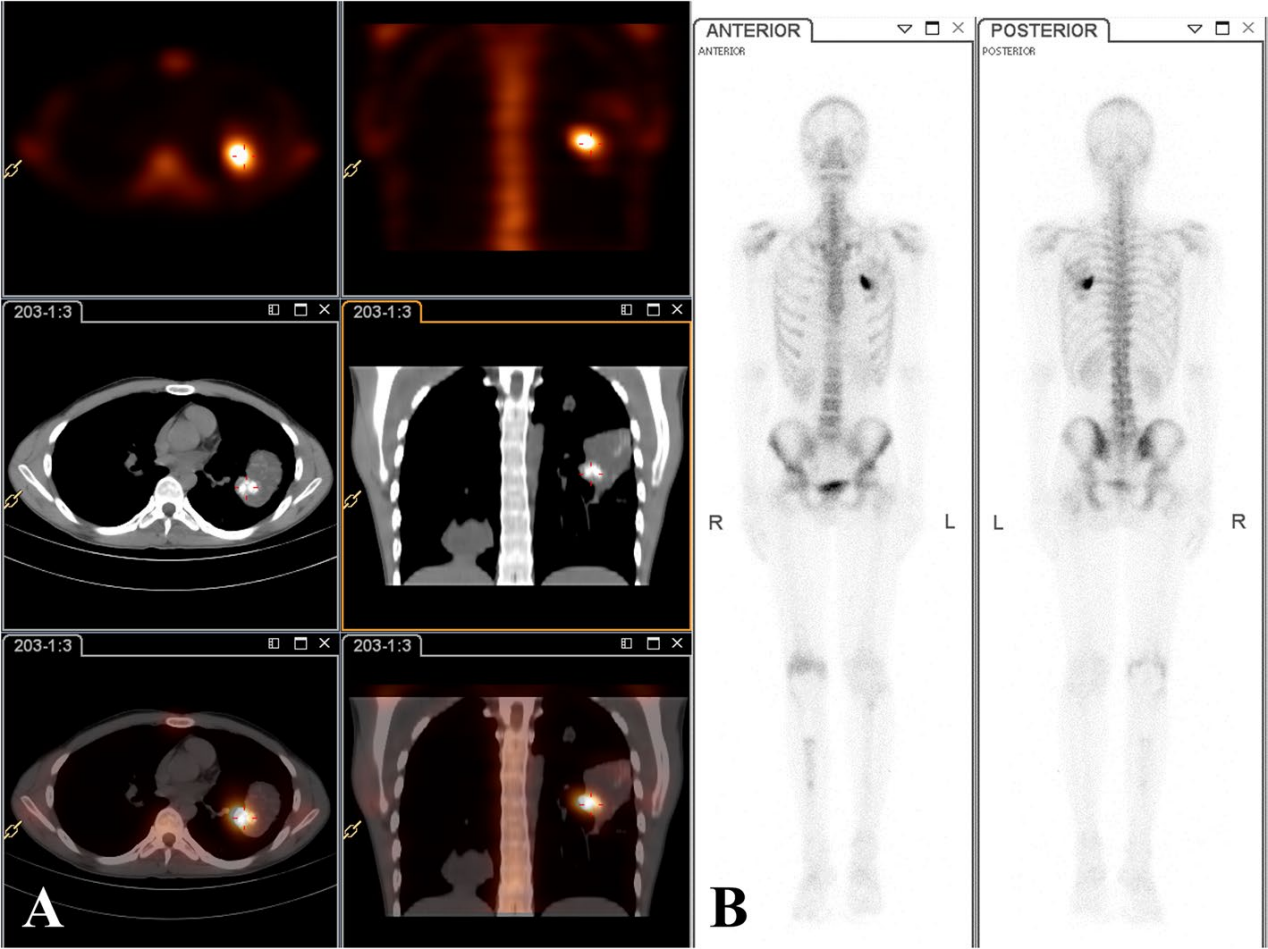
Single-photon emission computed tomography of the lung metastatic lesions in the bilateral lung. Single-photon emission computed tomography of the chest showed multiple pulmonary metastatic lesions in which the largest nodules in the left upper lobe with internal calcification (A). Bone scintigraphy showed increased uptake in the lesion of the left lung corresponding to the metastatic lesion (B)
